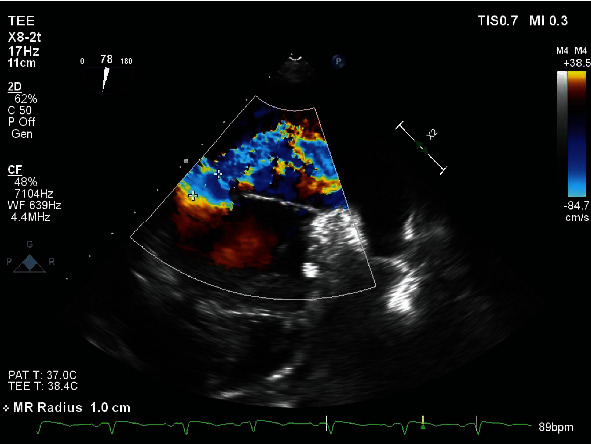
TEE with color Doppler showing severe eccentric mitral regurgitation.
